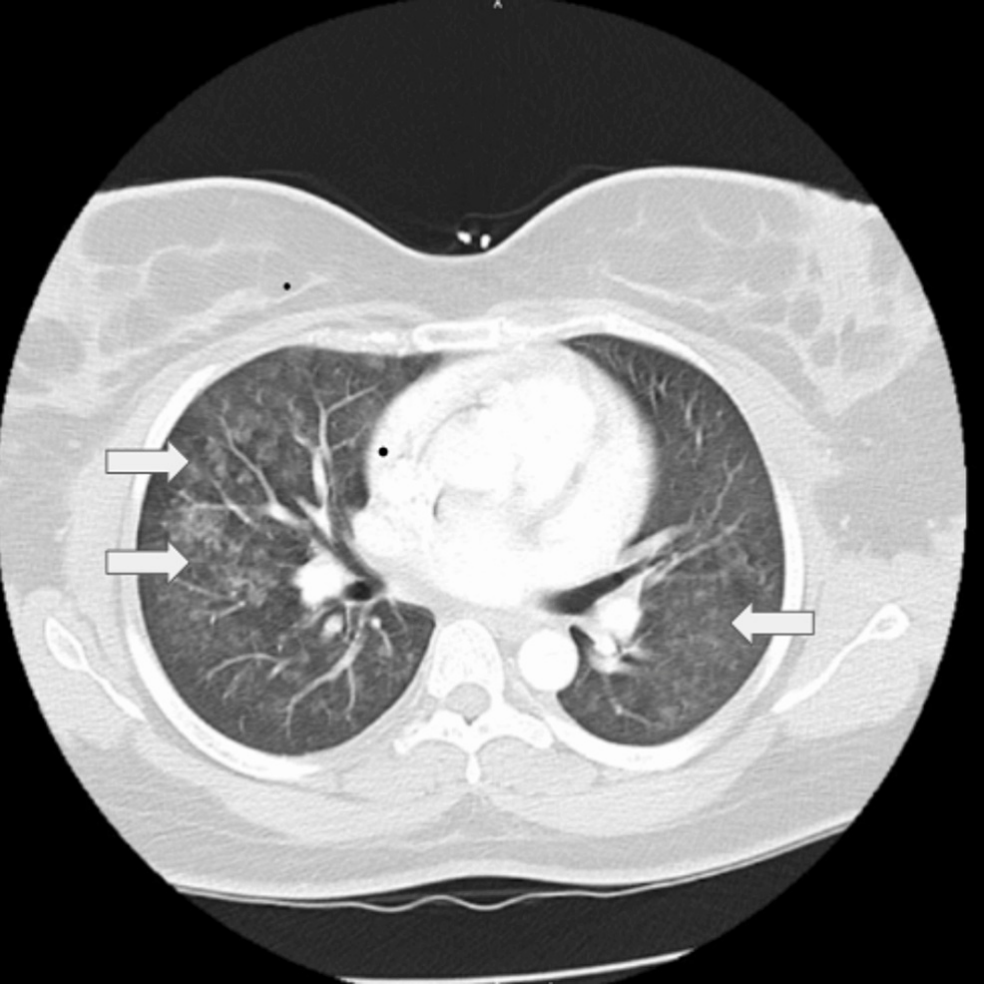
Computed tomography of the chest revealing bilateral diffuse centrilobular nodular ground-glass opacities
